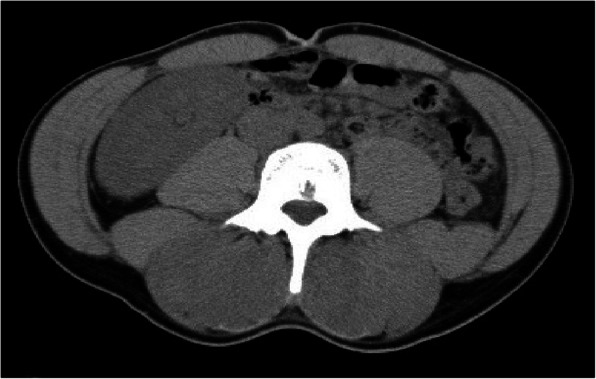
Abdominal computed tomography at admission. Swelling was noted in the paravertebral muscle
